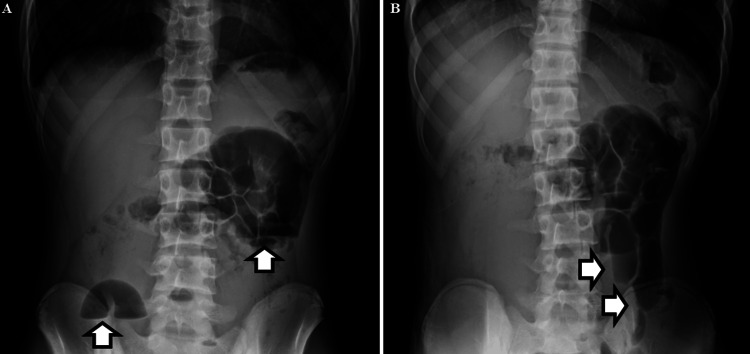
Plain abdominal radiographs that were obtained at initial presentation. (A) Upright view shows diffuse bowel gas distension with multiple air-fluid levels (arrows). (B) Right lateral decubitus view demonstrating marked intestinal gas accumulation without evidence of mechanical obstruction, consistent with paralytic ileus (arrows).
